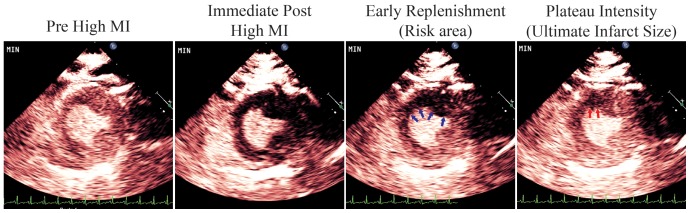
Myocardial Perfusion Images. Myocardial perfusion images from the low MI contrast imaging that were used to guide the application of the therapeutic ultrasound (TUS) impulses. Note that after the TUS impulses, there was no myocardial contrast present in the left circumflex or left anterior descending perfusion beds (Second panel from the left). During low MI triggered imaging, early replenishment delineated the risk area (arrows; third panel from the left). A plateau intensity was reached (right panel), which was when the TUS impulses were re-applied.
